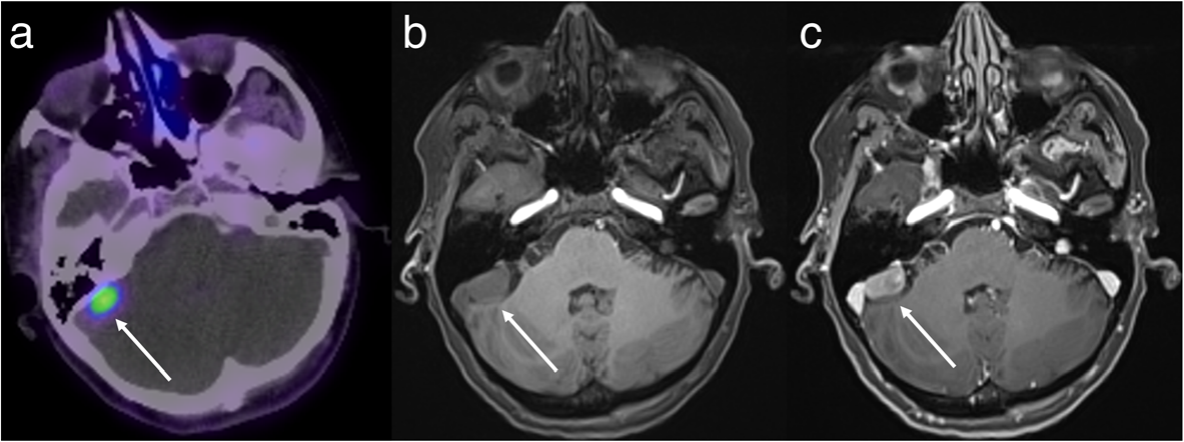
GaTate-PET (a) reveals a right posterior fossa mass (arrow). The pre- and post-contrast MRI images (b and c, respectively) demonstrate a homogeneously-enhancing durally-based mass, consistent with a meningioma. This diagnosis was also supported by evidence of calcification on CT (not shown)
